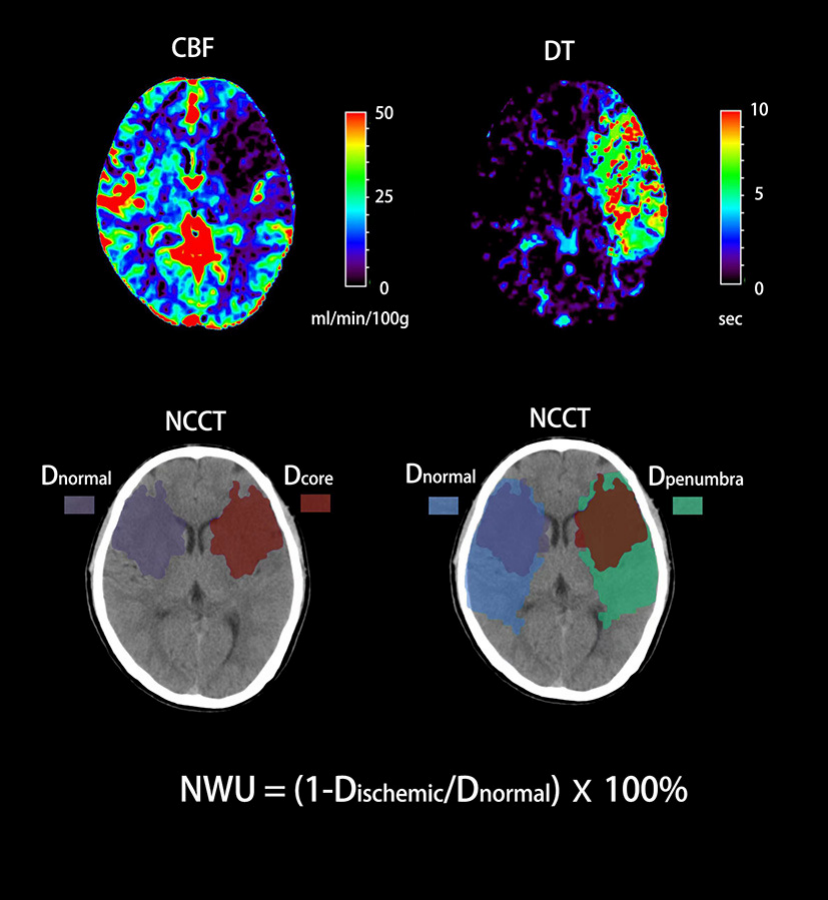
Quantification of NWU-core and NWU-penumbra in admission CT. CBV and DT maps based on CTP were used to identify the ischemic core and penumbra with the setting of different thresholds. The mean density of the ischemic core, ischemic penumbra, and normal tissue derived from mirrored contralateral were then calculated (*D*_r*e*_, *D*_p*enra*_, and *D*_n*ra*_).
